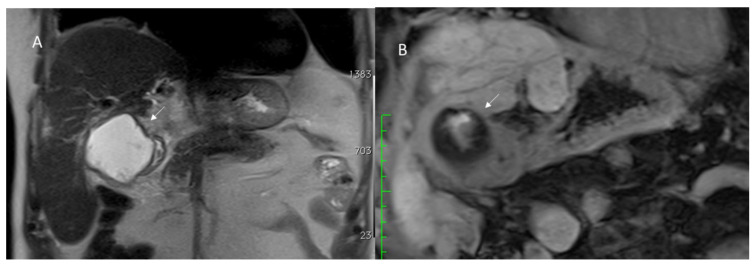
Bile leaks assessed with MRI T2-W sequence (A) and hepatospecific phase of contrast study (B). Arrow shows leak.
